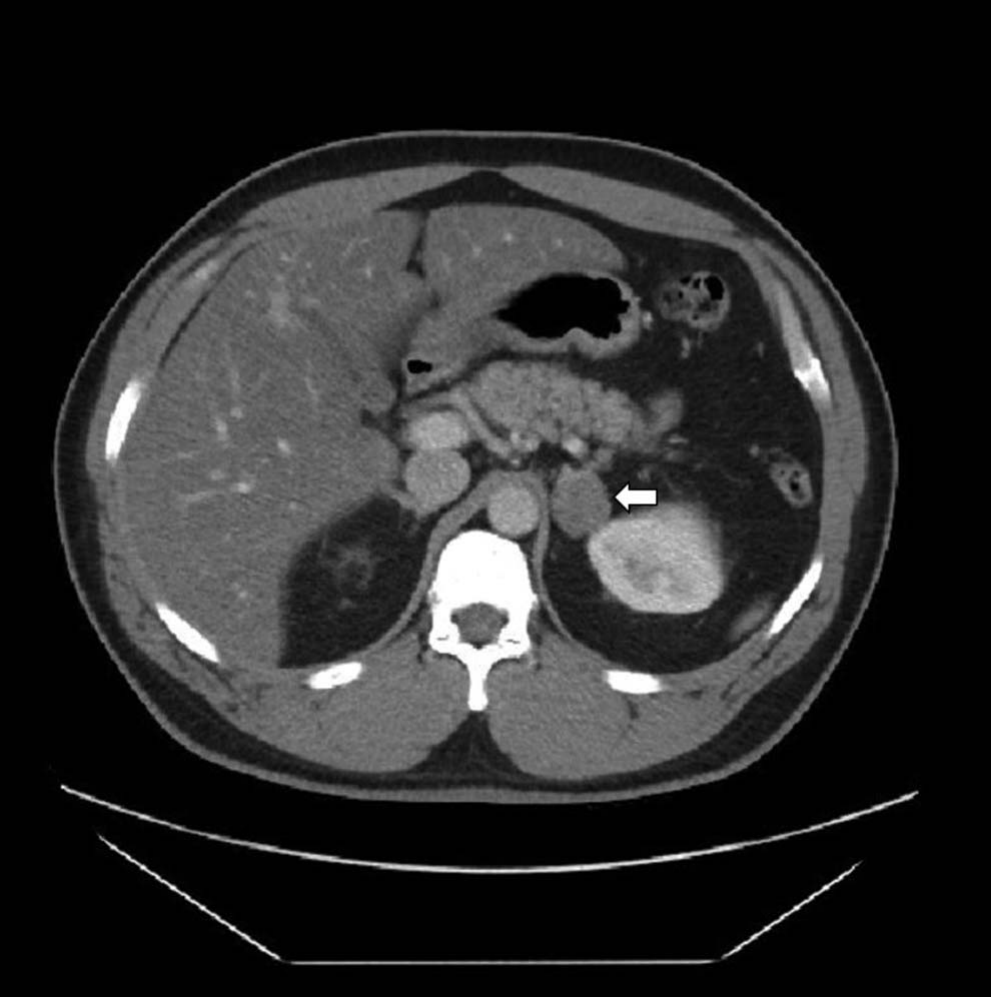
Computed tomography scan of abdomen and pelvis with contrast shows interval development of a 2.8-cm left adrenal nodule with imaging characteristics consistent with an adenoma (arrow).
